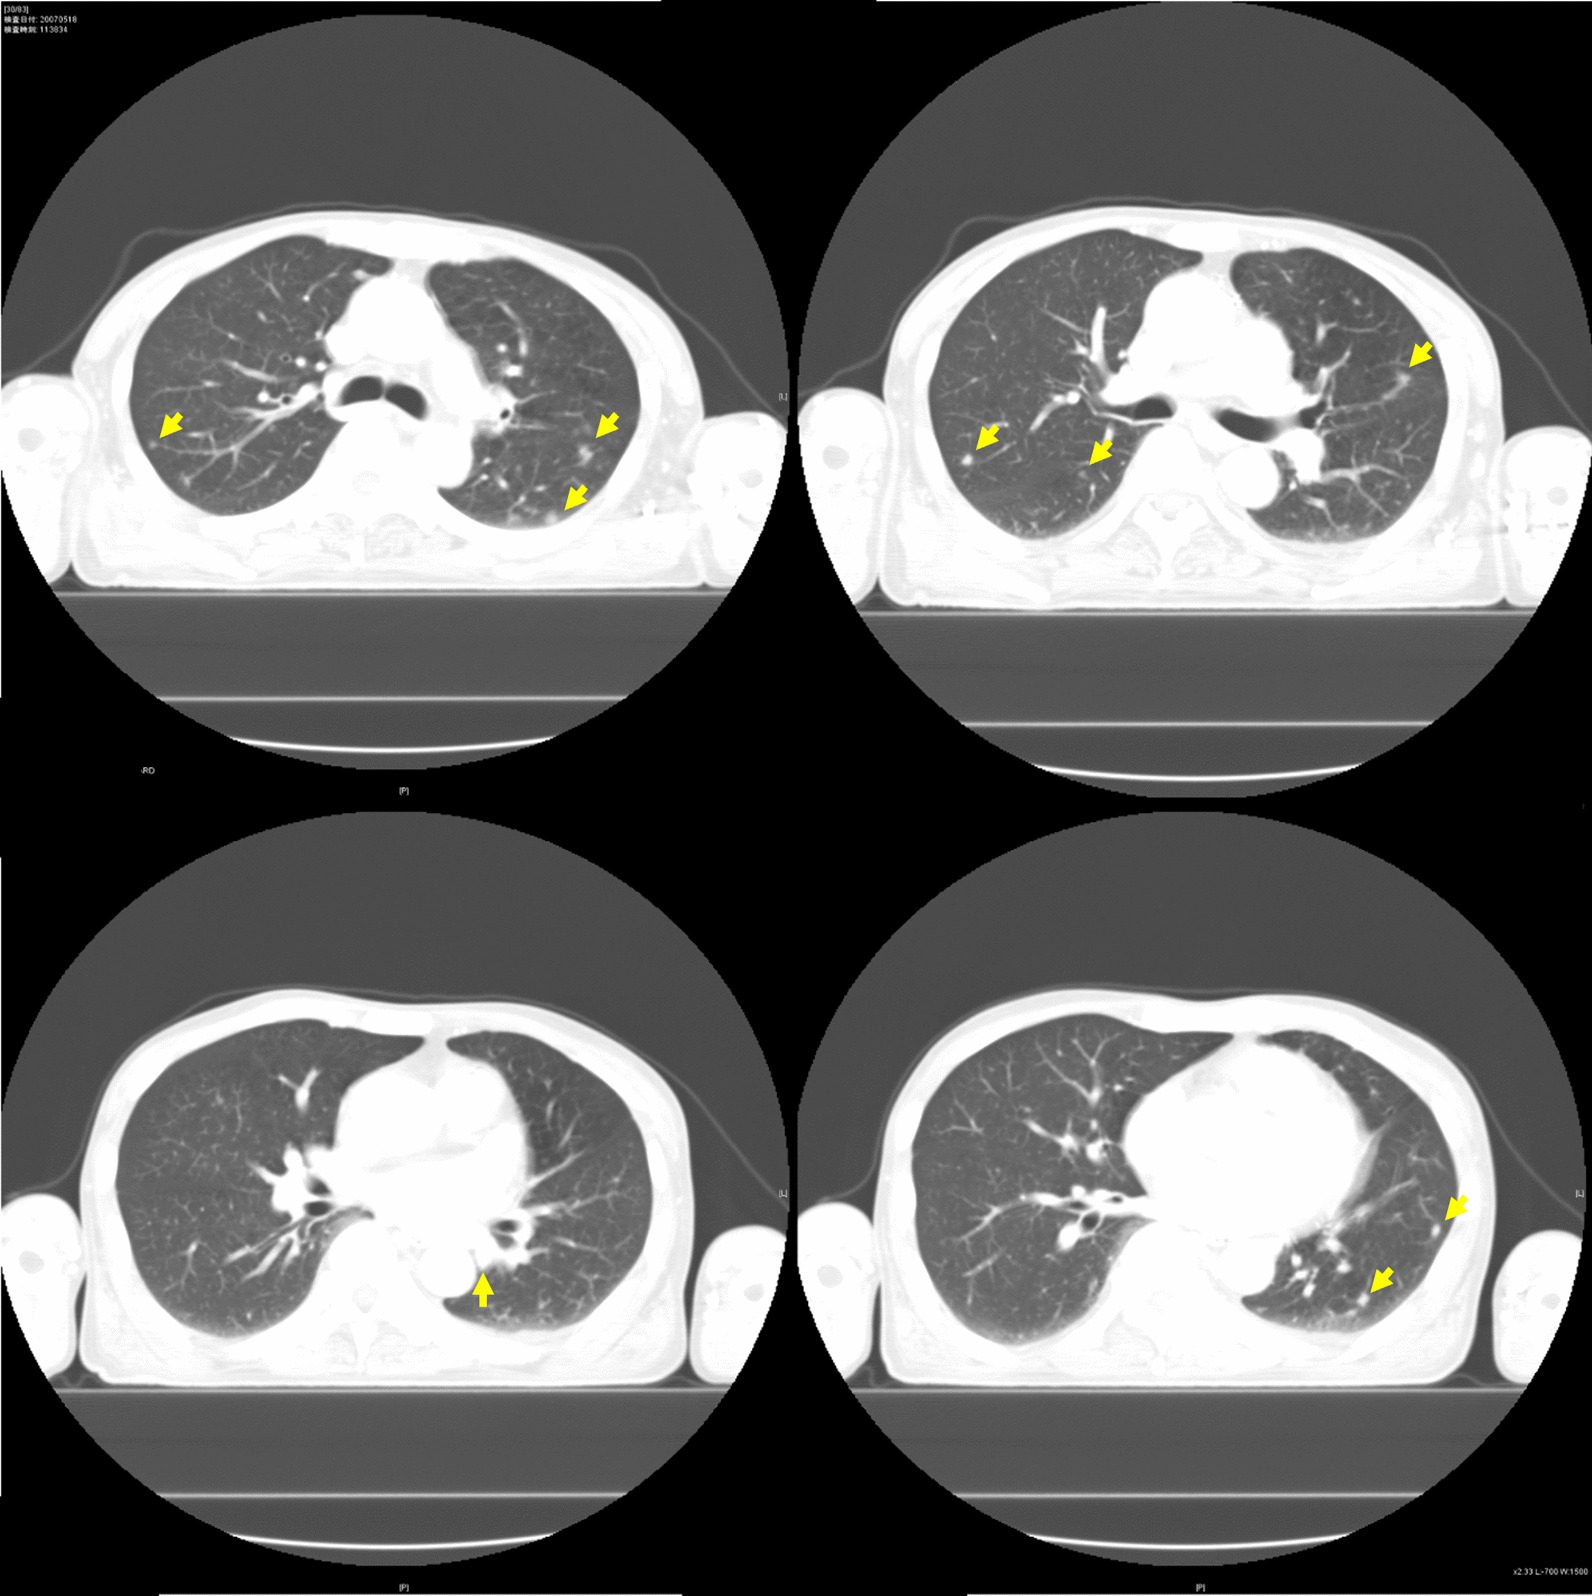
Computed tomography showed multiple nodules in bilateral lungs (yellow arrows)
